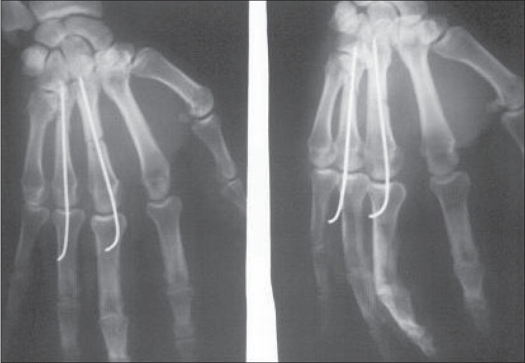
Postoperative radiographs showing good alignment after fixation with two Kirschner wires
